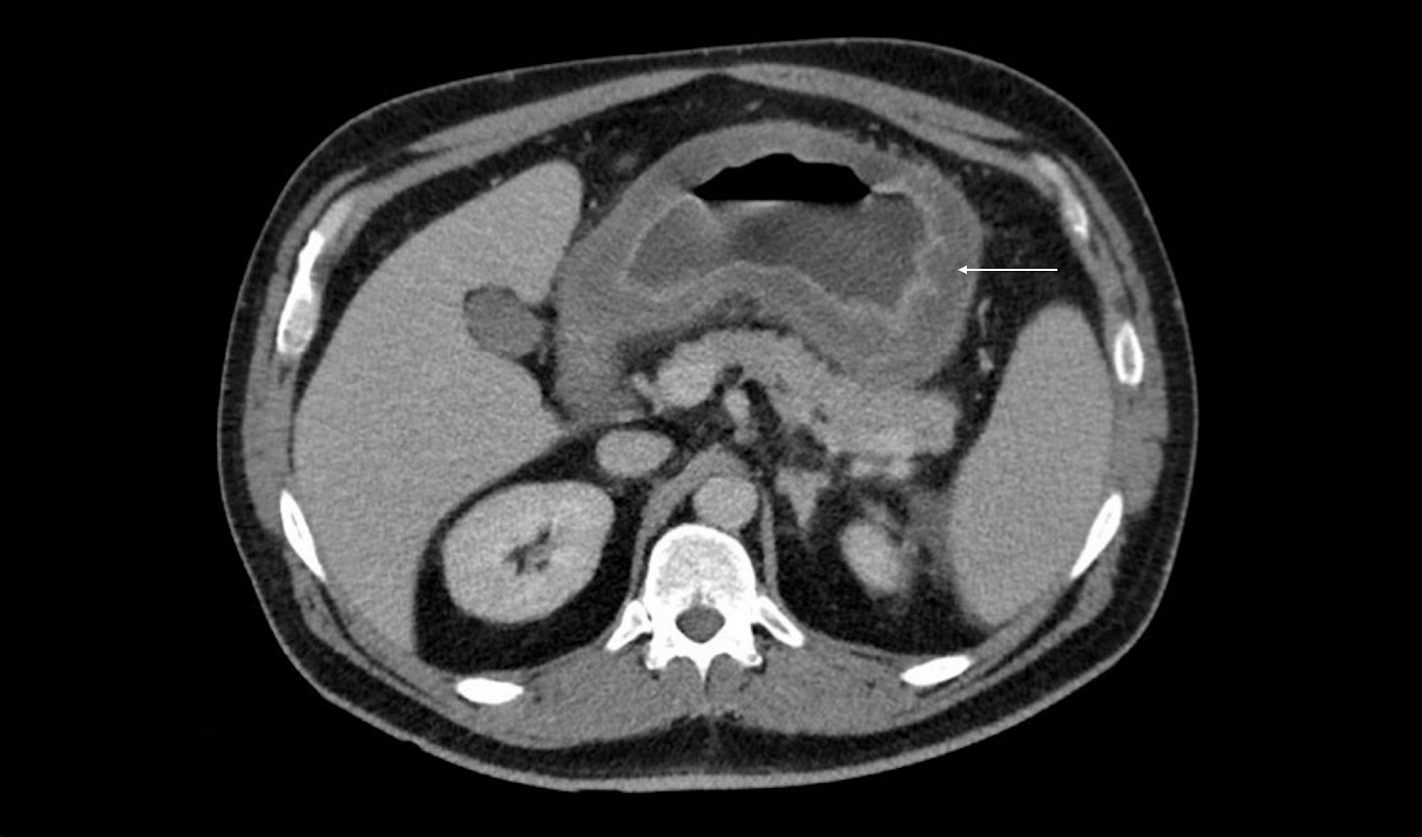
Contrast-enhanced abdominal CT demonstrating diffuse gastric wall thickening (up to 1.6 cm) with mucosal enhancement CT: computed tomography
